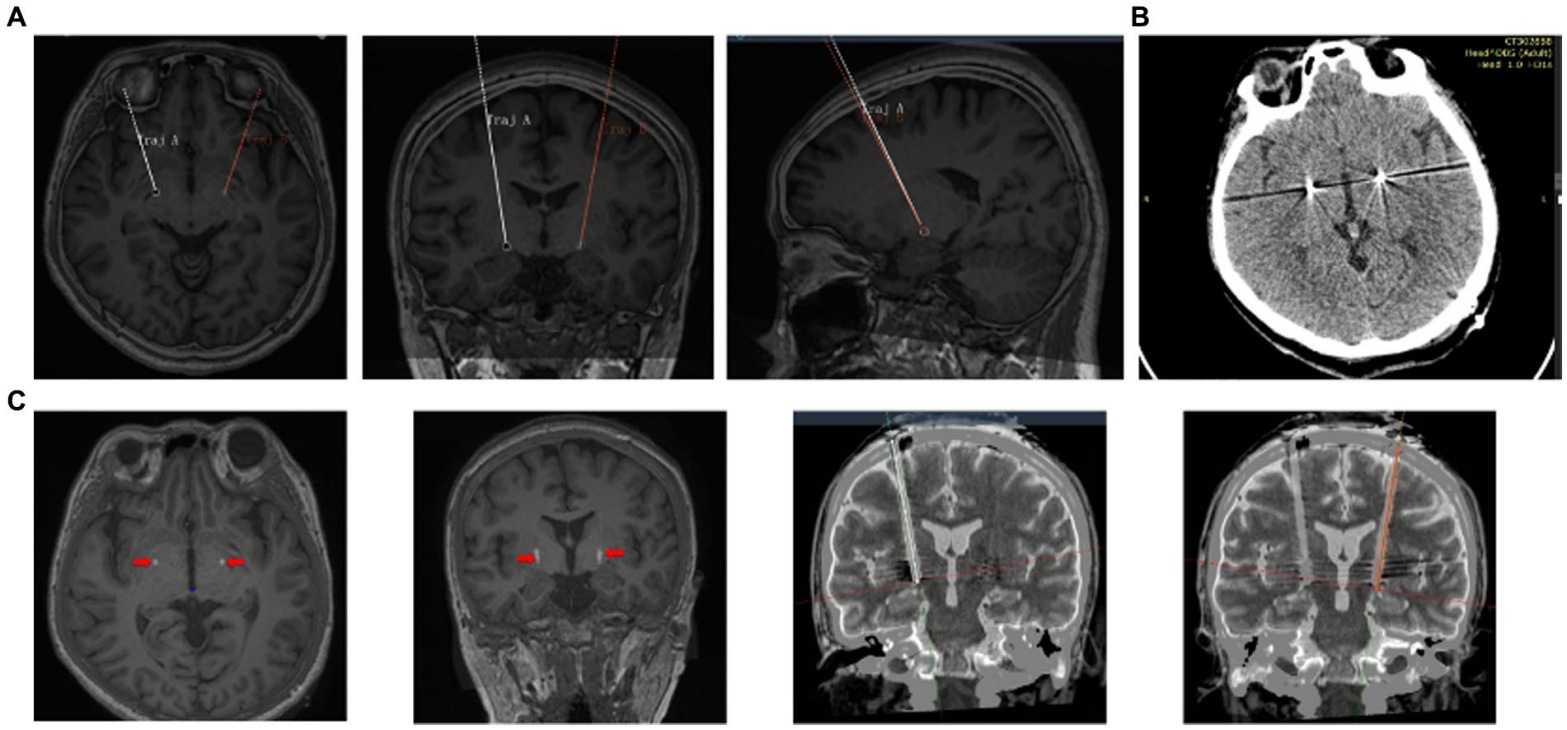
(A) Preoperative craniocerebral 3.0 T MRI and skull CT data with frame were imported into Surgiplan software to develop the surgical plan. (B) CT scan after operation showed no bleeding or bubble around the electrodes. (C) Postoperative CT and preoperative MRI fusion showed that electrode implantation trajectory and implantation position were accurate.
